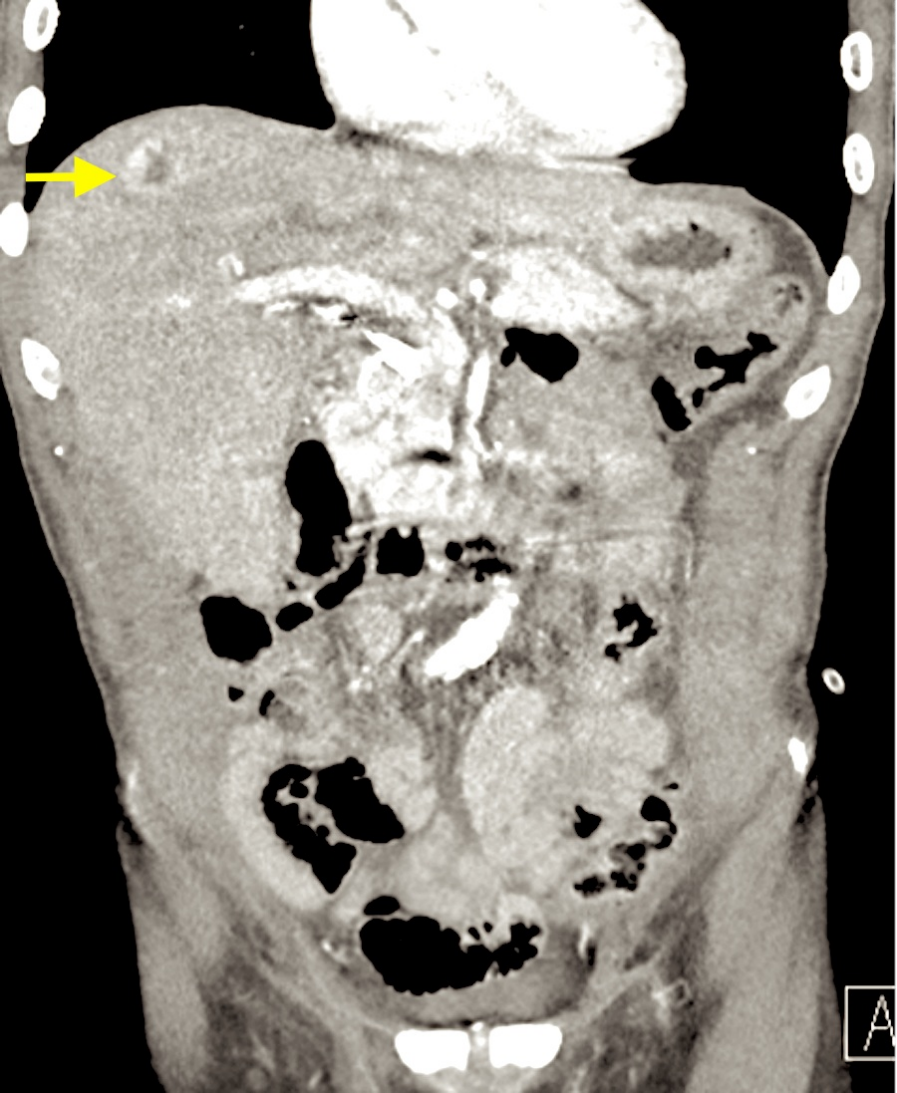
CT scan showing intensely enhancing mass in segment 8 of the liver (yellow arrow)
